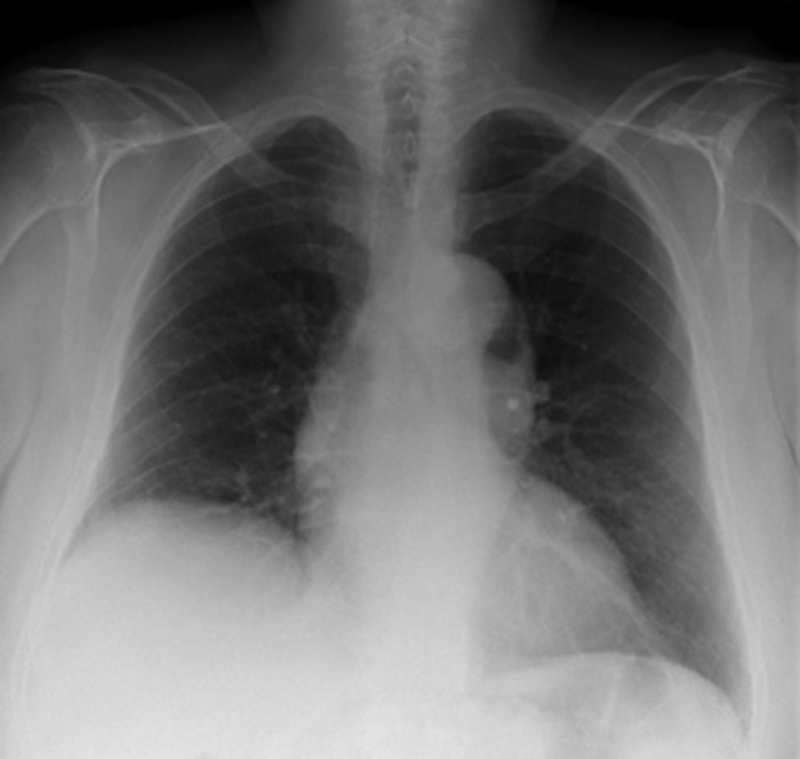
Anteroposterior chest radiograph demonstrating a widened mediastinum.
